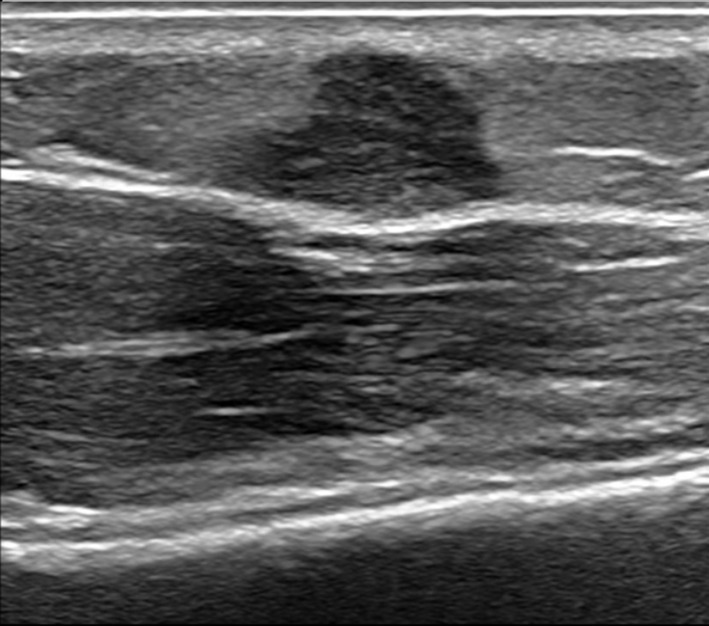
An ultrasound examination of the left deltoid nodule at the vaccination site revealed a lobulated, hypoechoic lesion measuring 0.8 cm.
